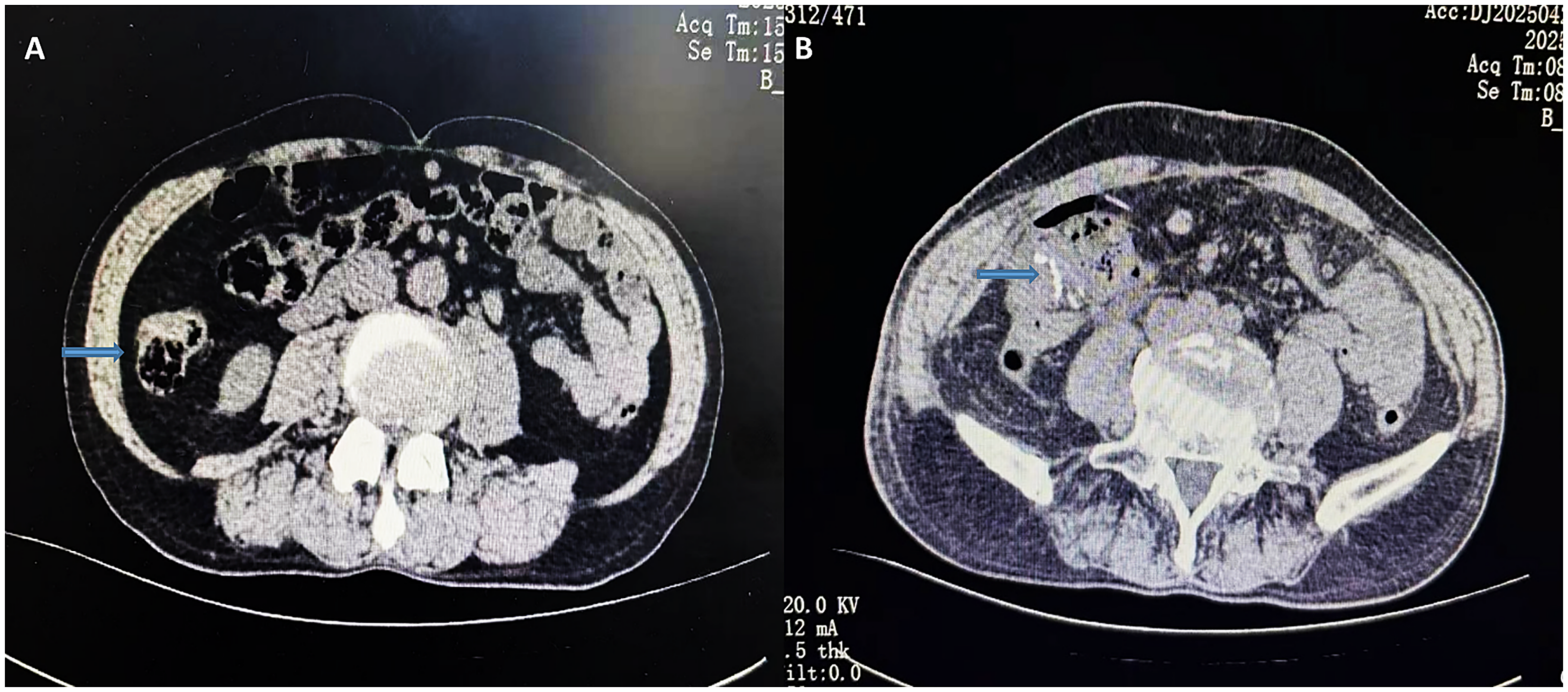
Preoperative CT transverse section of the abdomen (A); postoperative CT transverse section of the abdomen (B). The arrows indicate preoperative localized bowel wall thickening and the postoperative anastomosis.
